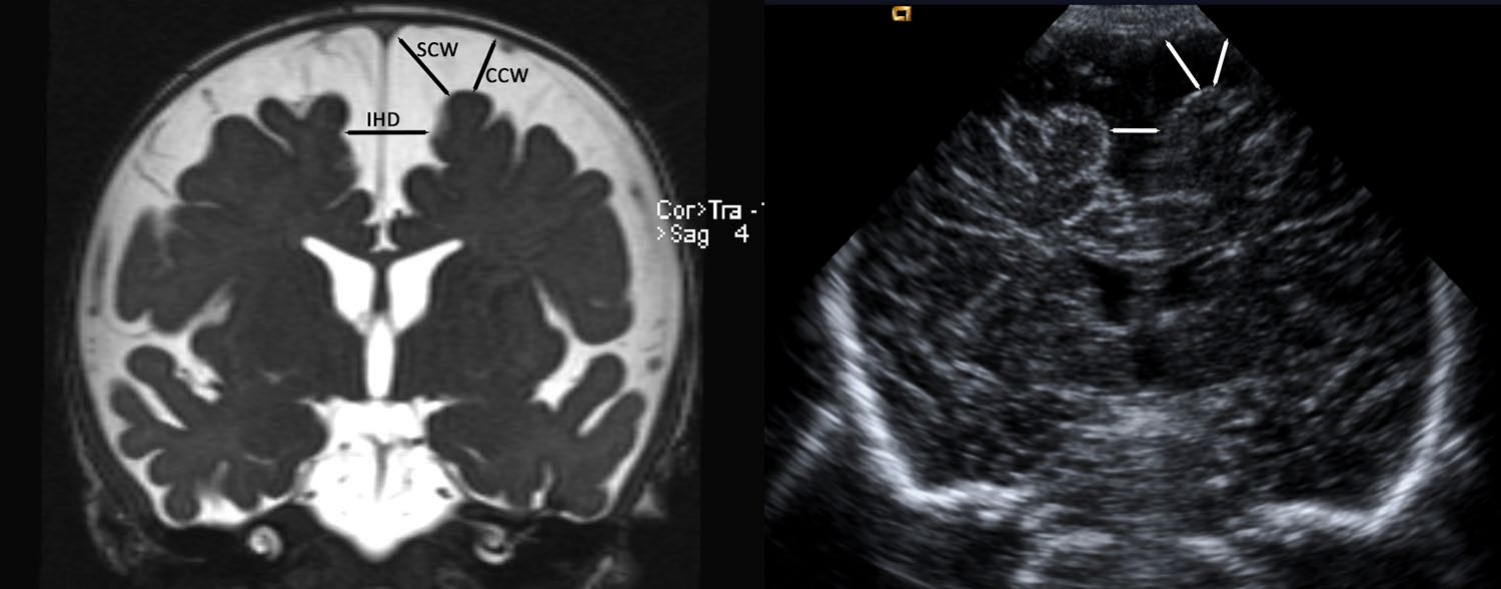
Radiological parameters to assess CSAS width on MRI and US scans from the same boy at an age of 5.5 months, when he had developed macrocephaly 2 cm > P97 and very pronounced subarachnomegaly. The underlying cause was a high-grade bilateral transverse sinus stenosis. A coronal T2-weighted MRI scan (left) and a coronal transfontanellar ultrasound scan (right) are shown. The craniocortical width (CCW), the sinocortical width (SCW), and the interhemispheric distance (IHD) are shown correspondingly in MRI and ultrasound
